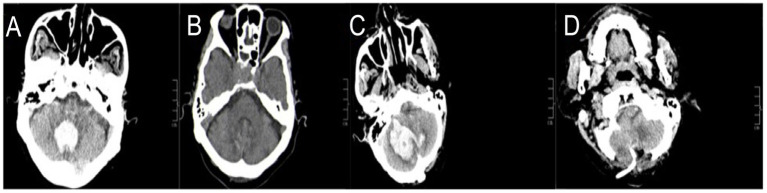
(A–D) The results of brain computed tomography scan before and after surgical treatment.
